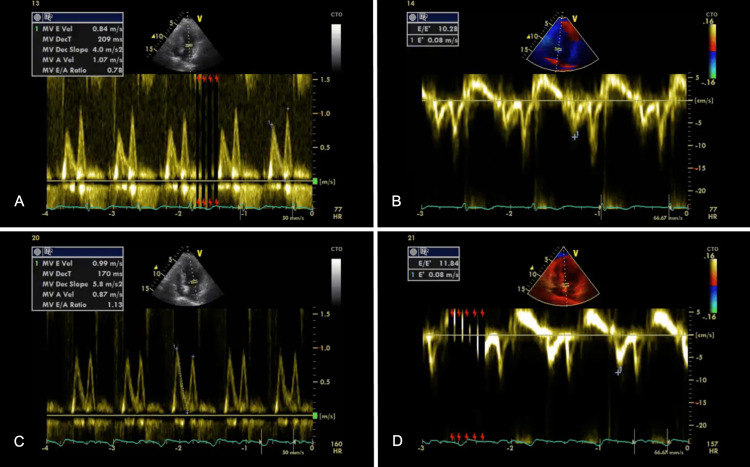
(A and B) - differences in mitral valve and tissue Doppler findings obtained on a subsequent time lapse of four days. On admission, depicting indices; MV E Velocity= 0.84 m/s, MV A Velocity= 1.07 m/s, MV E/A Ratio= 0.78, E/E’ Ratio= 10.28 suggestive of grade I diastolic dysfunction. (C and D) - Pre-Discharge, depicting indices; MV E Velocity= 0.99 m/s, MV A Velocity= 0.87 m/s, MV E/A Ratio= 1.13, E/E’ Ratio= 11.84 suggestive of grade II diastolic dysfunction.
